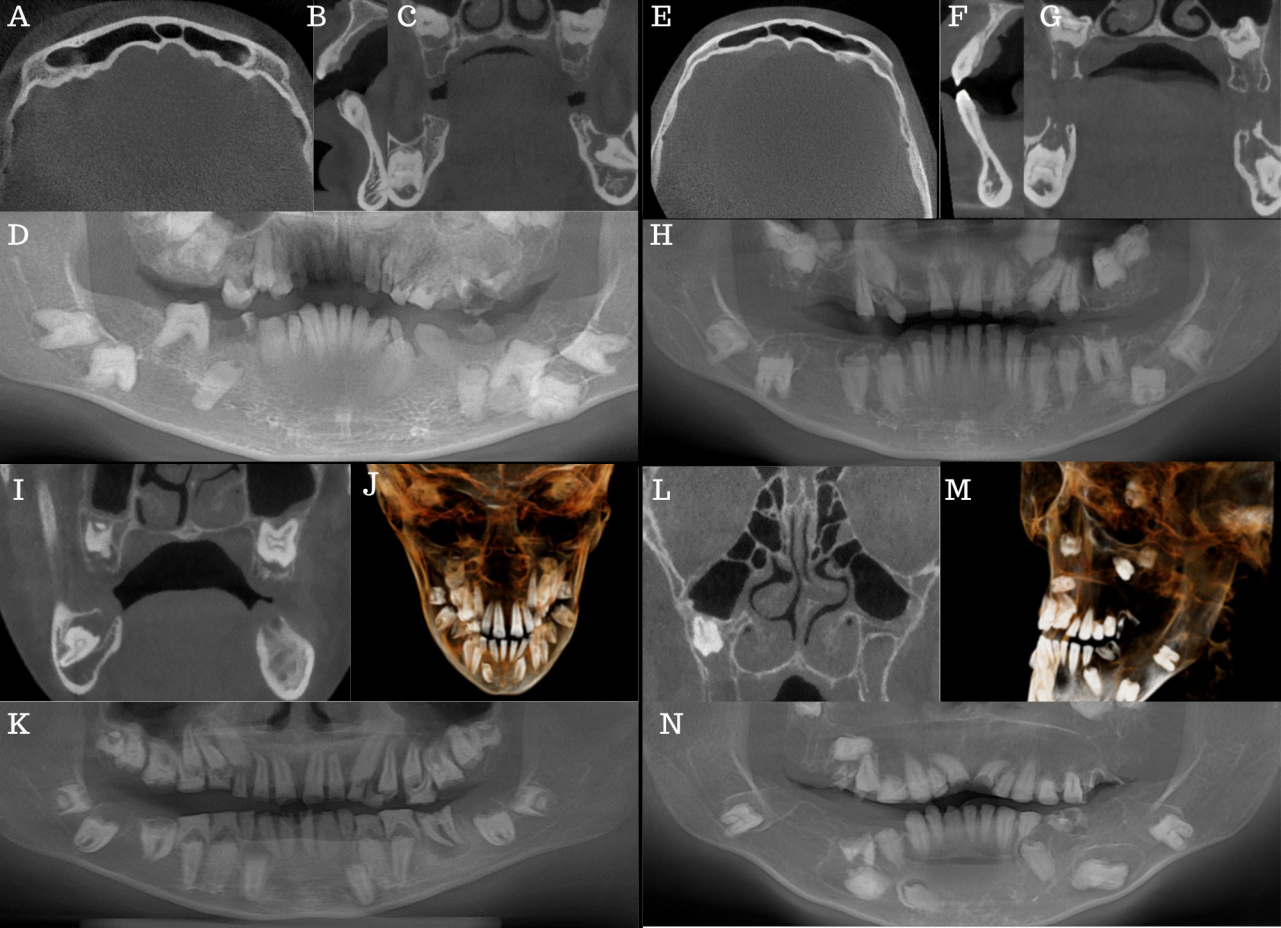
Case 1* (A–D): (A) axial view showing frontal sinus pneumatisation. (B) Sagittal view showing severe thinning of the lower anterior alveolar ridge. (C) Coronal view showing several impactions, pulp stones, and enlarged dental follicles stars. (D) CBCT reformatted panorama. *Case 2* (E–H): (E) Axial view showing frontal sinus pneumatisation. (F) Sagittal view shows severe lower and upper anterior alveolar ridge thinning. (G): Coronal view showing several impactions, pulp stones and enlarged dental follicles stars. (H) CBCT reformatted panorama. Case 3 (I–K): (I) Coronal view showing several impactions, pulp stones, and enlarged dental follicles. (J) centre 3D model of the patient. (K) CBCT reformatted panorama. *Case 4* (L–N): (L) Coronal view showing hypoplastic maxillary antra. (M) Left sides of the 3D model of the patient. (N) CBCT reformatted panorama.
